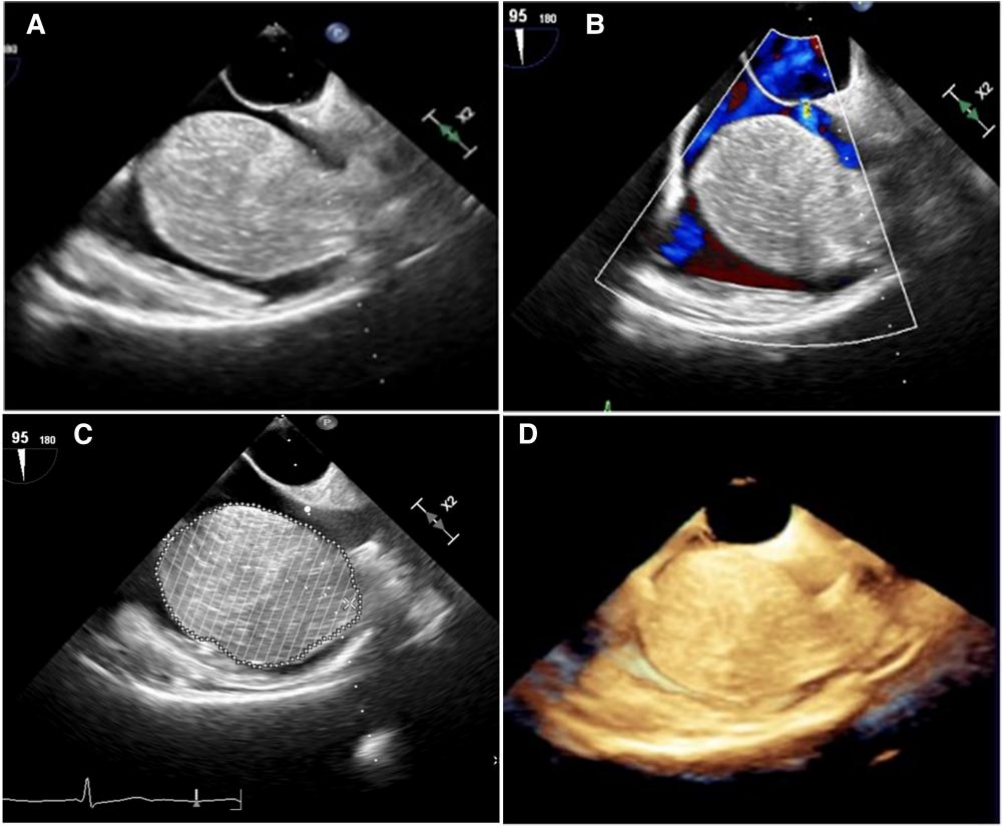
Transoesophageal echocardiography. (*A*) Combined bicaval view. (*B*) Combined bicaval view depicting opened PFO interatrial shunt, (*C*) measurement of tumour volume by Simpson method—136 mL, (*D*) 3D visualization. Own study.
